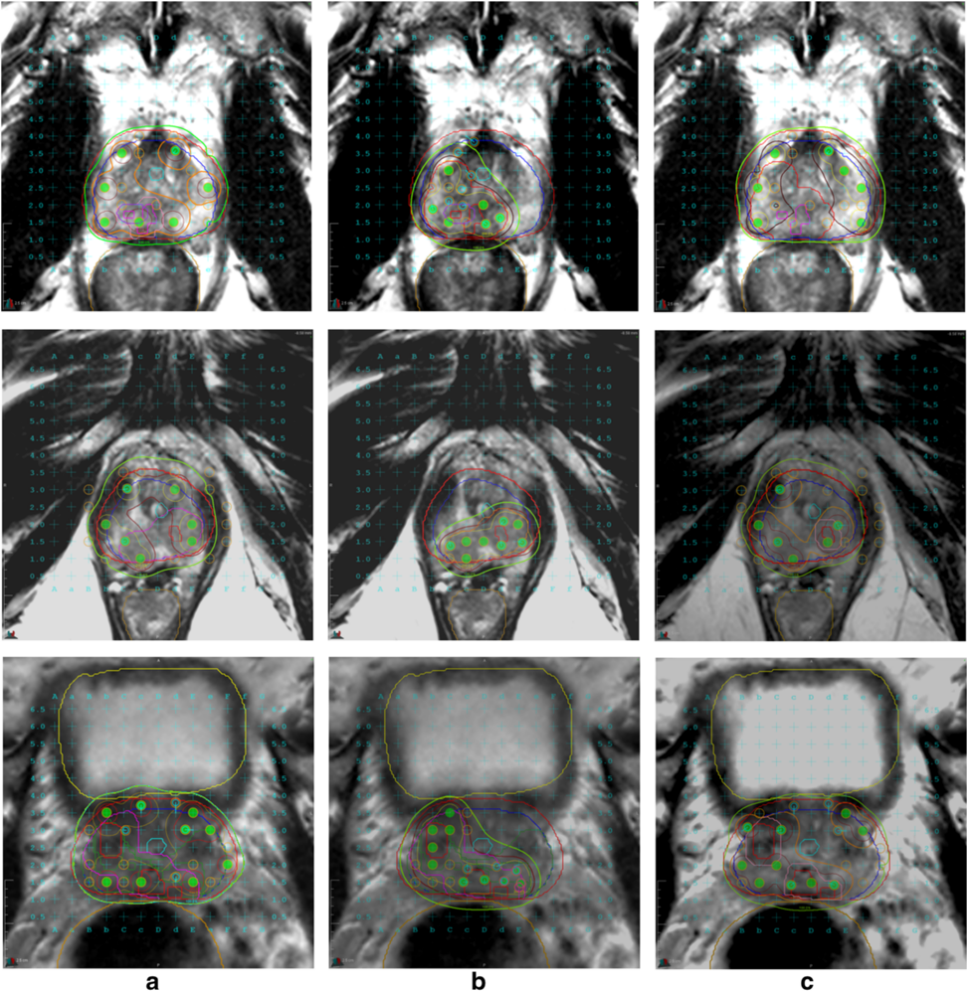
Different treatment plans for brachytherapy shown on a single slice of T2w MRI for 3 different patients (rows) - a
